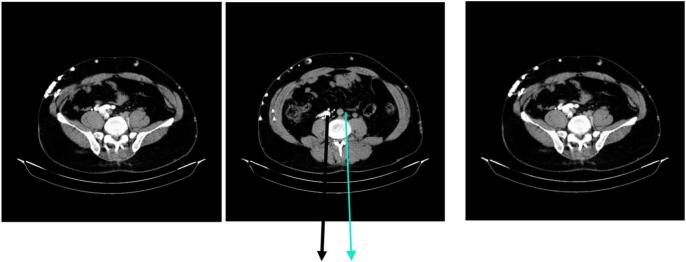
Computed tomography scans of case 2, green arrow indicating aorta, as the black one indicates an abnormal collateral vein. (For interpretation of the references to color in this figure legend, the reader is referred to the web version of this article.)
